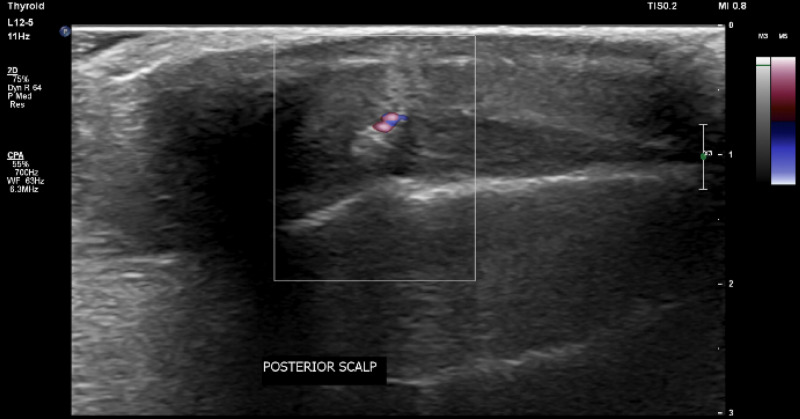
Hyperechoic region indicative of infestation with live botfly larva as evidenced by vascularity on doppler and movement on cine images. There is edema of the adjacent subcutaneous tissues with no sonographic evidence of abscess.
